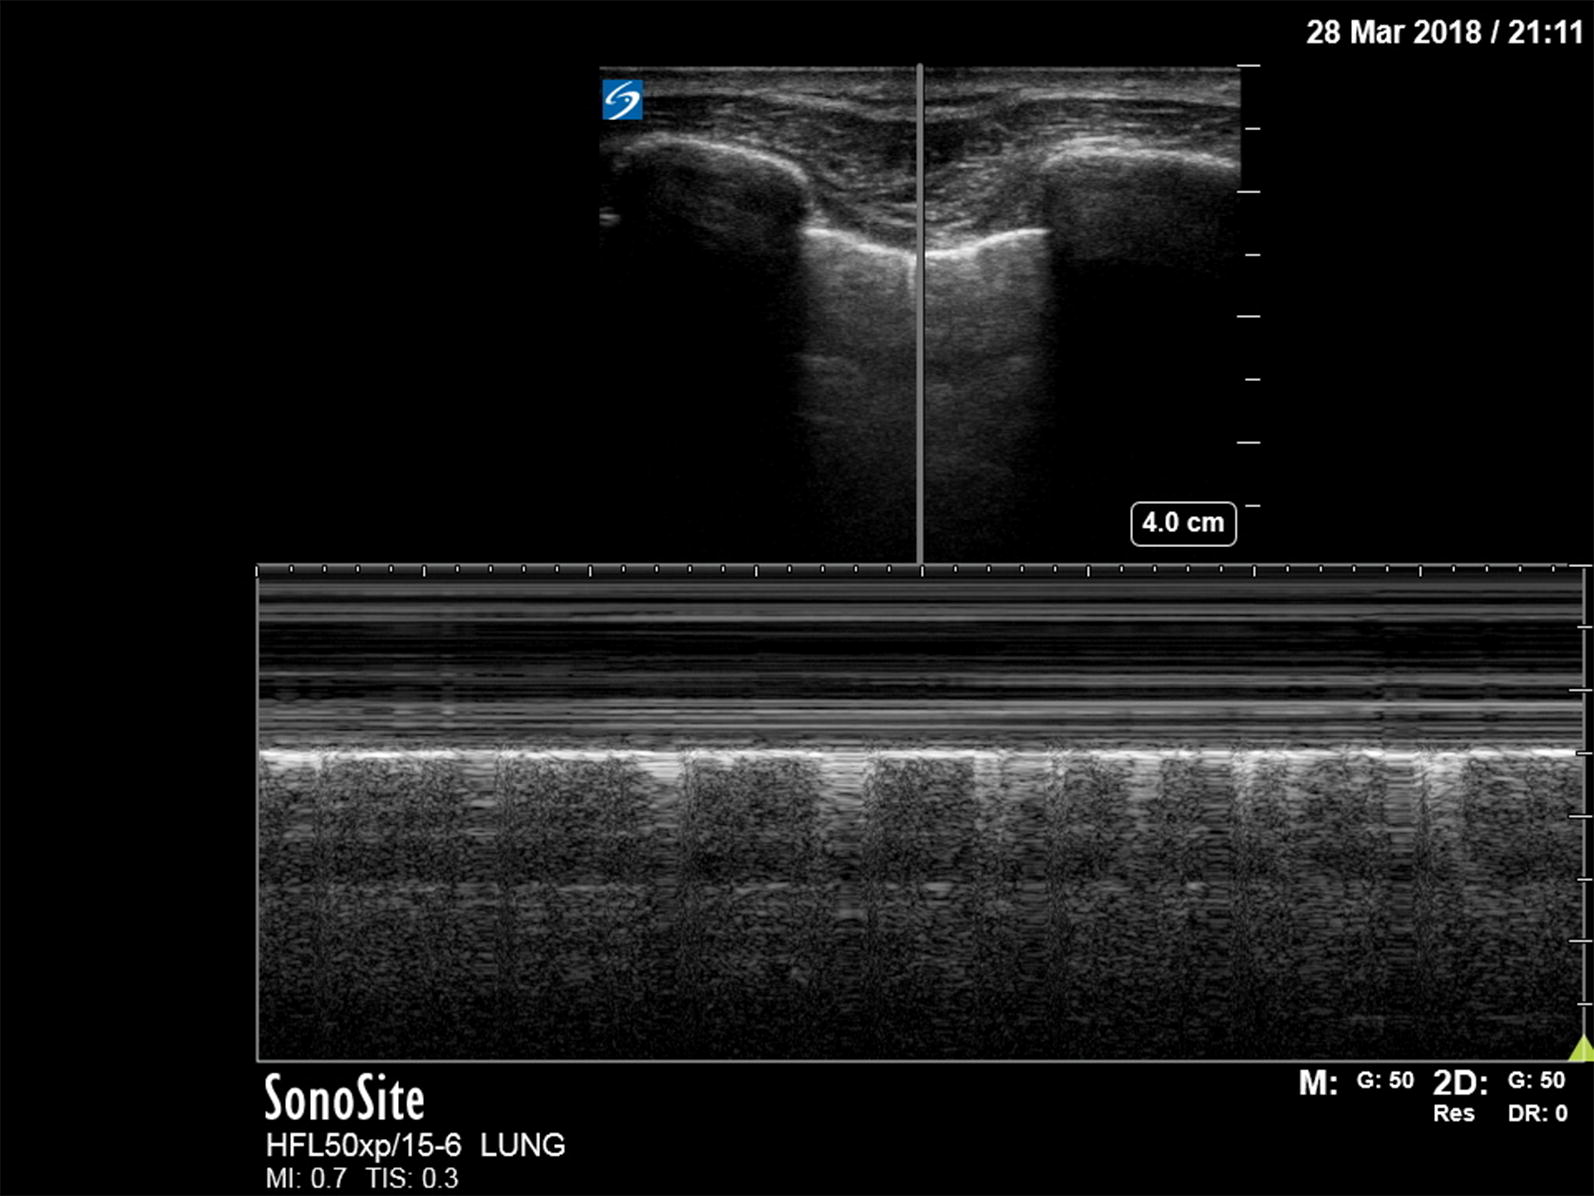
Normal lung—M-mode ultrasound image. The ultrasound reflections on the vertical line in the upper part of the image are sequentially displayed from left to right in the lower part as time progresses. It allows to capture the motion of the upper 2D image in the stationary image below. A normal M-mode image of the chest wall and pleura is displayed here. The stationary chest wall produces straight horizontal lines above the pleural line. The lung sliding and movement of the artifacts below produces a grainy image. This is called the seashore sign
